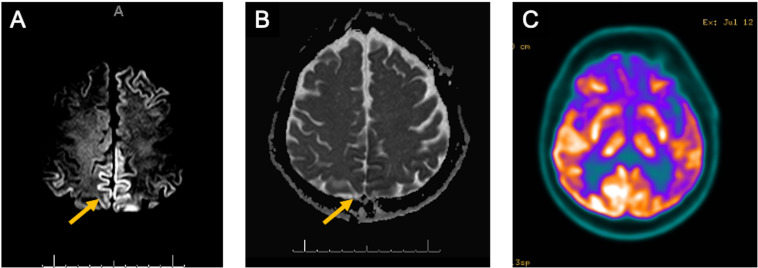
(A) MRI diffusion-weighted images from JJN (case 2) revealing hyperintensities on frontal, temporoparietal and posterior cingulate cortical areas (A, yellow arrow); (B) MRI apparent diffusion coefficient maps with corresponding hypointensities in the same regions above (B, yellow arrow); (C) 18-FDG PET/CT shows hypometabolism on the bilateral temporoparietal cortex, posterior cingulate, precuneus, and caudate nucleus.
